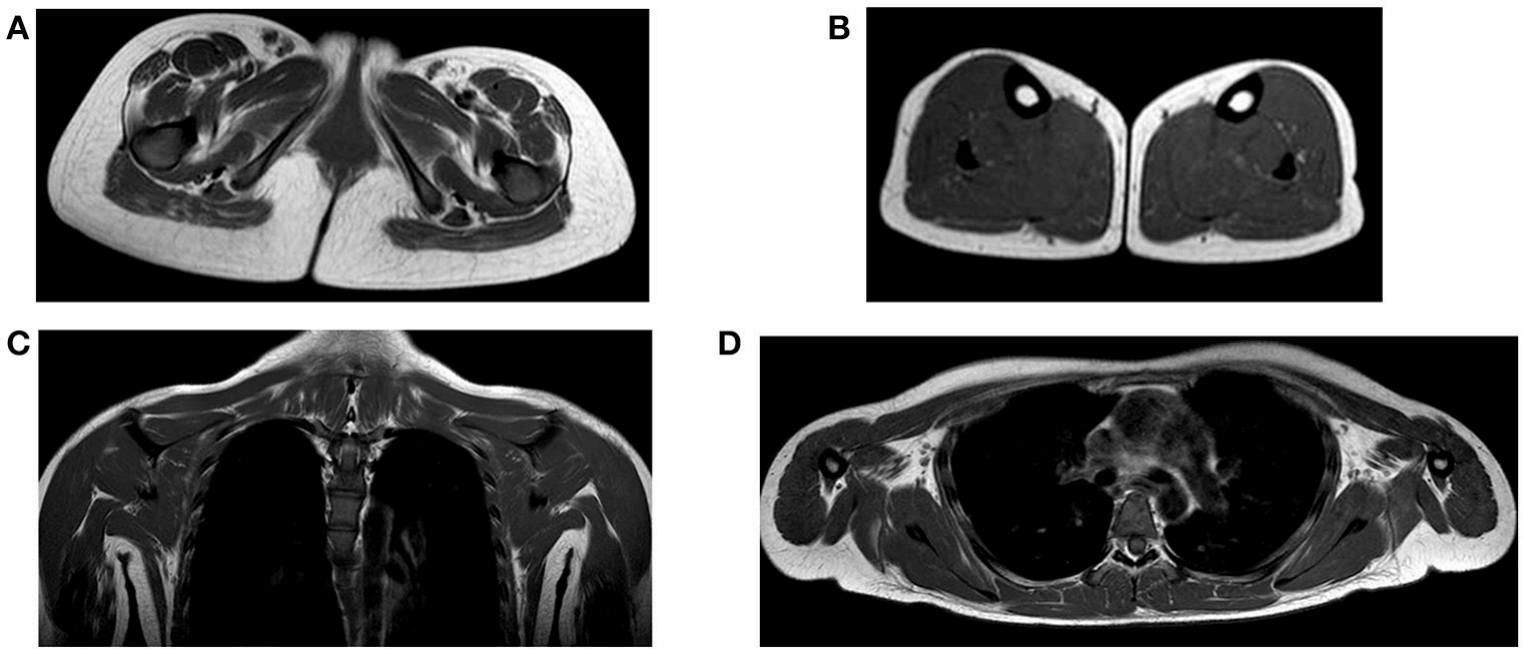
(A) Axial T1 image at the pelvis showing atrophy and moderate fatty replacement involving the gluteus maximus, particularly on the right side (black arrow); (B) axial T1 MR image at the level of the calf showing the relative sparing of the muscles with only moderate involvement of the gastrocnemii lateralis (dotted white arrow); (C,D) coronal and axial T1 MR images at the level of the cingular girdle showing mild fatty infiltration in the subscapularis muscles.
